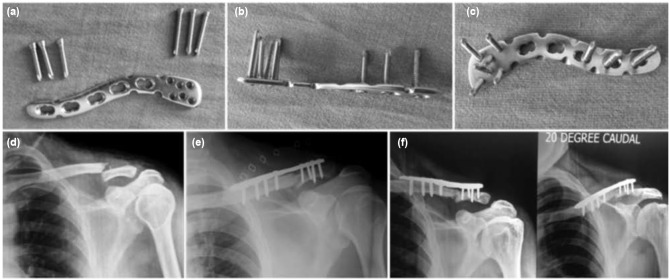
(a) Pre-contoured clavicular locking plate with broad lateral end along with small and large sized screws from superior surface, (b) from lateral surface, (c) from ventral surface, (d) Antero-posterior (AP) radiograph of shoulder joint showing lateral third clavicle fracture, (e) Immediate post-operative radiograph after fixation with locking plate and (f) AP and 20 degrees caudal view of clavicle 9 months after surgery showing union of fracture.
